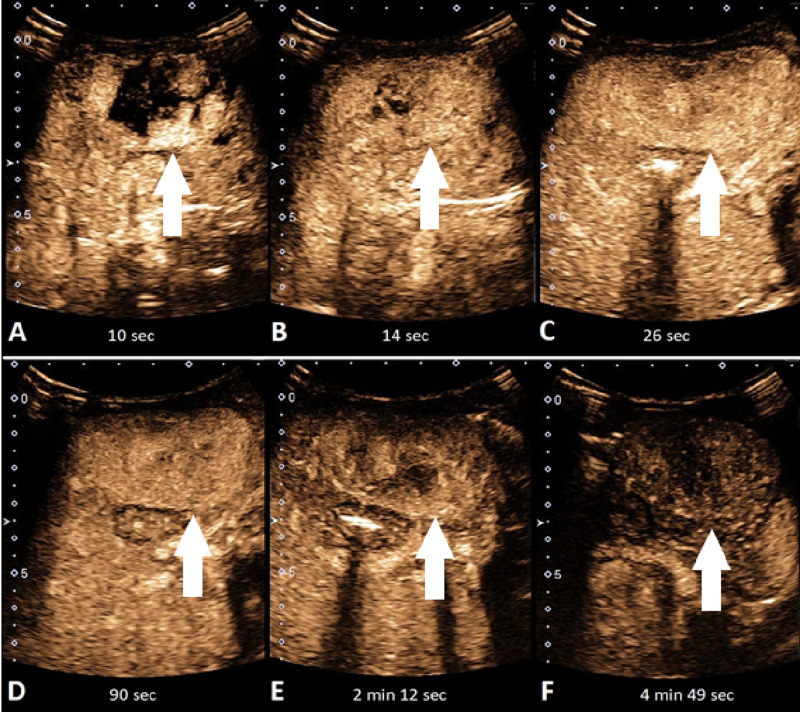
Abdominal contrast-enhanced ultrasound (CEUS) phases A liver CEUS at different times after IV contrast administration: (A) peripheral enhancement of the lesion after 10 seconds, (B, C) fast and complete filling of the lesion with contrast medium in the late arterial phase (14 and 26 seconds), (D-F) completely filled lesion with contrast medium remaining slightly hyperenhanced compared to normal liver tissue  in the venous and portal phase (90 seconds, 2 minutes and 12 seconds, 4 minutes and 49 seconds).
